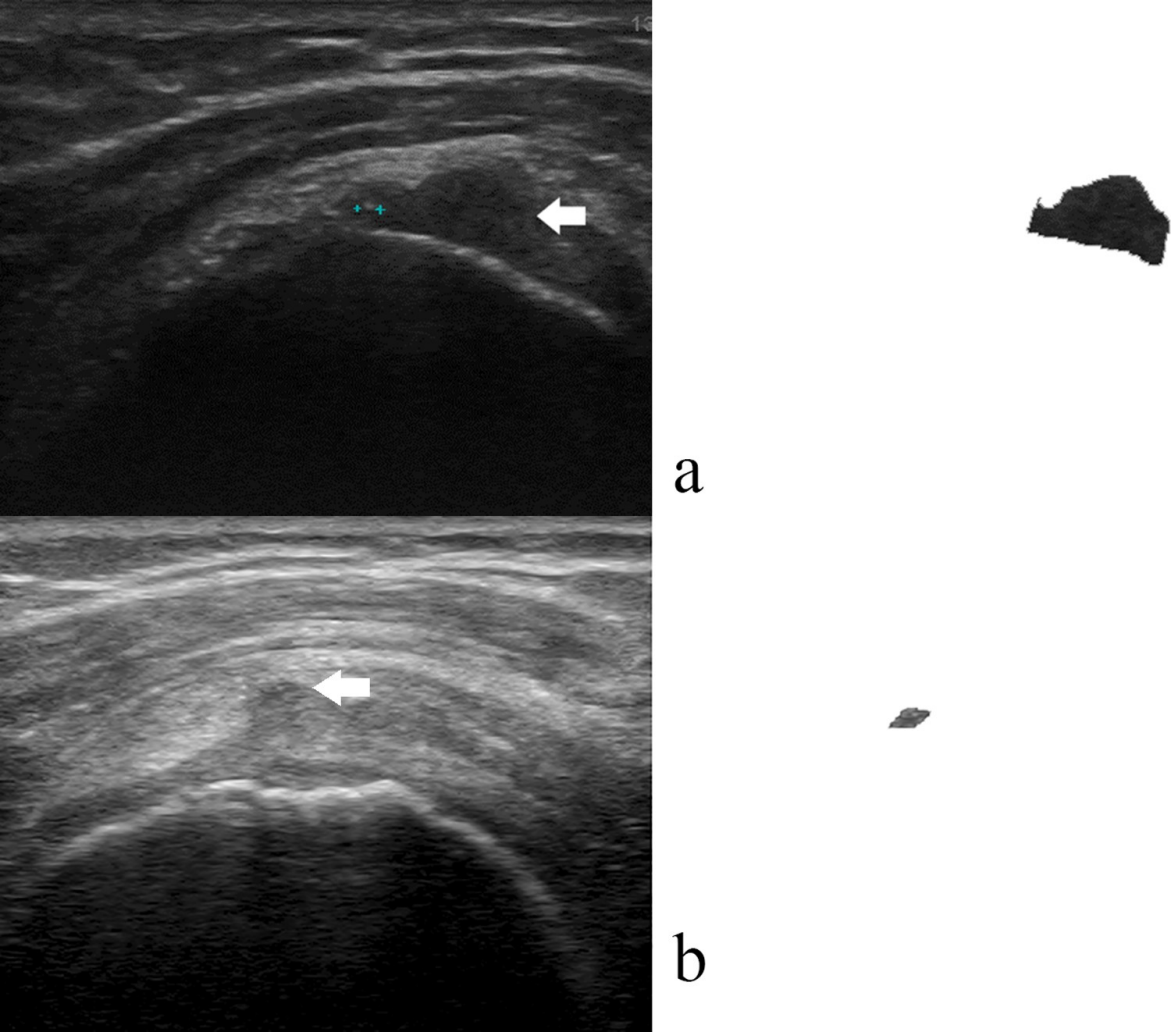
Tear classification results with probabilities higher than 50% were classified to be tear. (a) a moderate supraspinatus near full thickness tear with unobvious characteristics in the ultrasound image (hypoechoic area near the tendon insertion indicated by a white arrow) was misclassified by the texture feature set (42%) but correctly classified by the combination of texture and intensity feature sets (100%). (b) a small supraspinatus partial thickness tear at bursal surface was misclassified by the intensity feature set (9%) but correctly classified by the combination of texture and intensity feature sets (68%).
